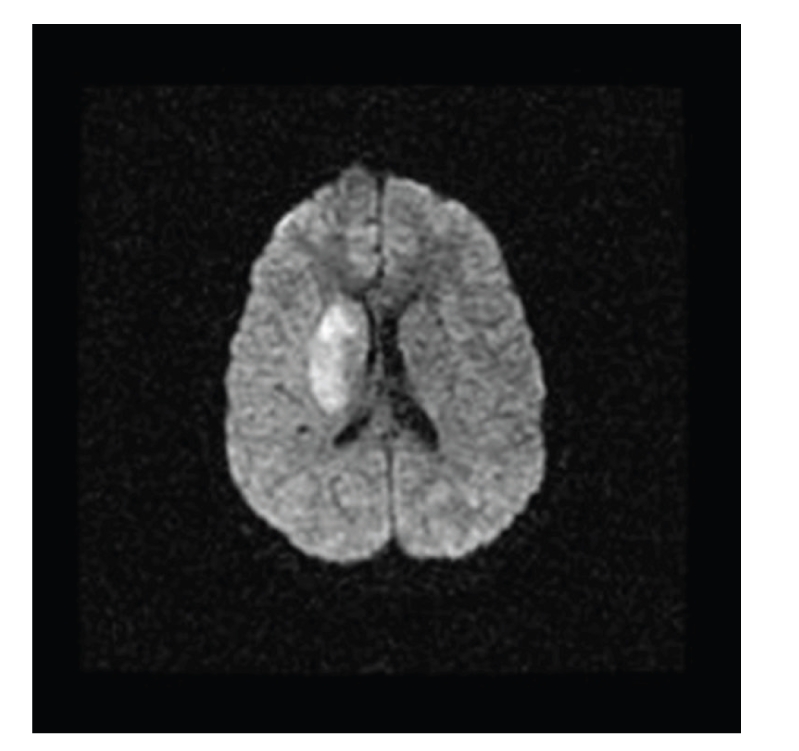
Diffusion weighted imaging (DWI) showing area of restricted diffusion.
